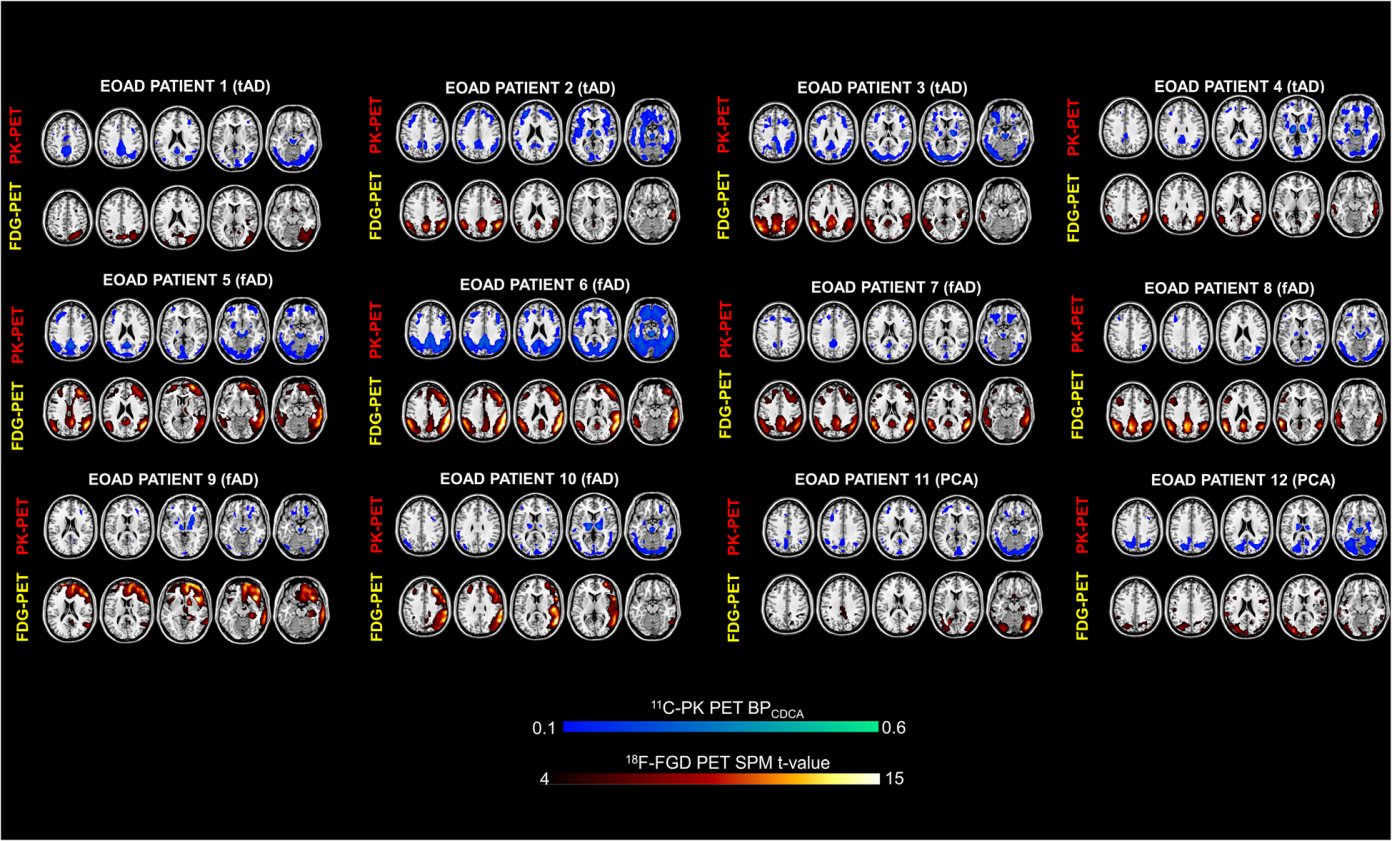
Patterns of [^18^F]-FDG PET brain hypometabolism and [^11^C]-PK 11195 PET binding potential in single individuals. tAD, typical Alzheimer disease; fAD, frontal AD variant; PCA, posterior cortical atrophy variant. Color scales for FDG and PK levels of significance
